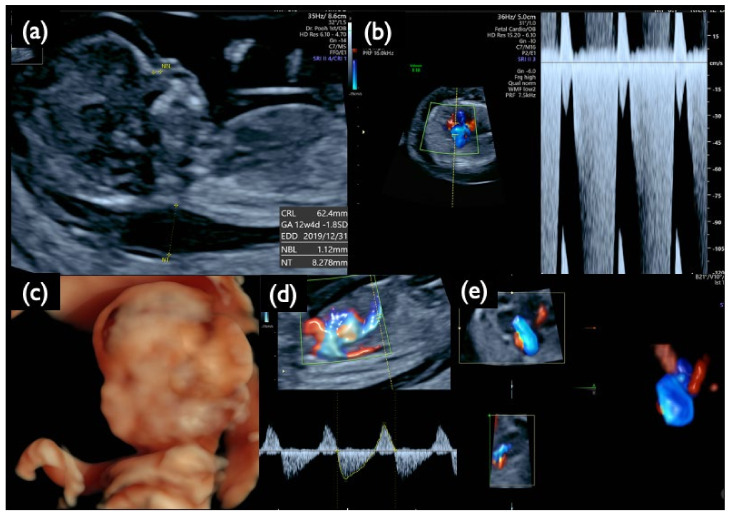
Sonographic findings at 13 weeks and 5 days of gestation in Case Mo5 with T18 mosaicism and a negative CRITO-NIPT result. (a) Mid-sagittal section of the fetal head. Nuchal translucency (NT) of 8.3 mm, a small nasal bone, and micrognathia are demonstrated. (b) Severe tricuspid regurgitation blood flow. (c) 3D reconstructed image of fetal face and upper extremities. Micrognathia, a low-set ear, and wrist contracture are visualized. (d) Reversed end-diastolic flow of ductus venosus. (e) 4D cardiosonographic image. Cardiomegaly with outflow tract abnormality is demonstrated. Intrauterine fetal demise was confirmed thereafter.
